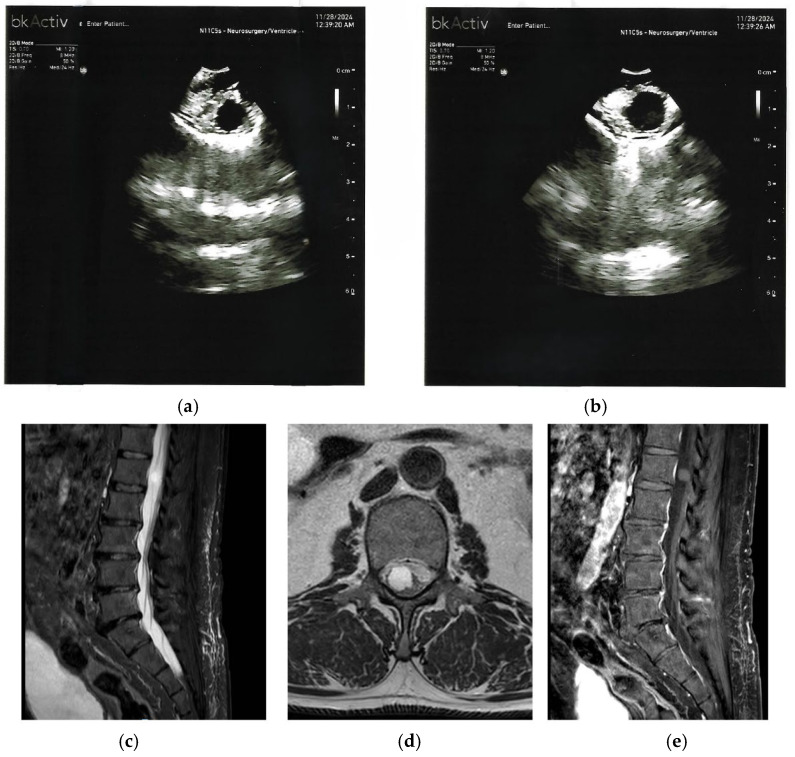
(a,b) Pre-durotomy intraoperative ultrasound shows a thin-walled, anechoic cyst (arrow in (a) indicates fine internal septation), producing mass effect with eccentric displacement of the spinal cord (labeled ‘cord displacement’ in (b)). The anechoic cavity on IOUS (a,b) corresponds to the CSF-like signal on MRI (c,d). (c) Sagittal T2-weighted MRI demonstrates a well-circumscribed intradural, extramedullary cystic lesion with CSF-like signal and focal cord compression (arrow indicates compression). (d) Axial T2-weighted MRI at the level of maximal compression confirms an intradural cyst eccentrically displacing the cord (labeled ‘cord displacement’). (e) Sagittal post-contrast T1-weighted MRI shows no enhancing solid component.
